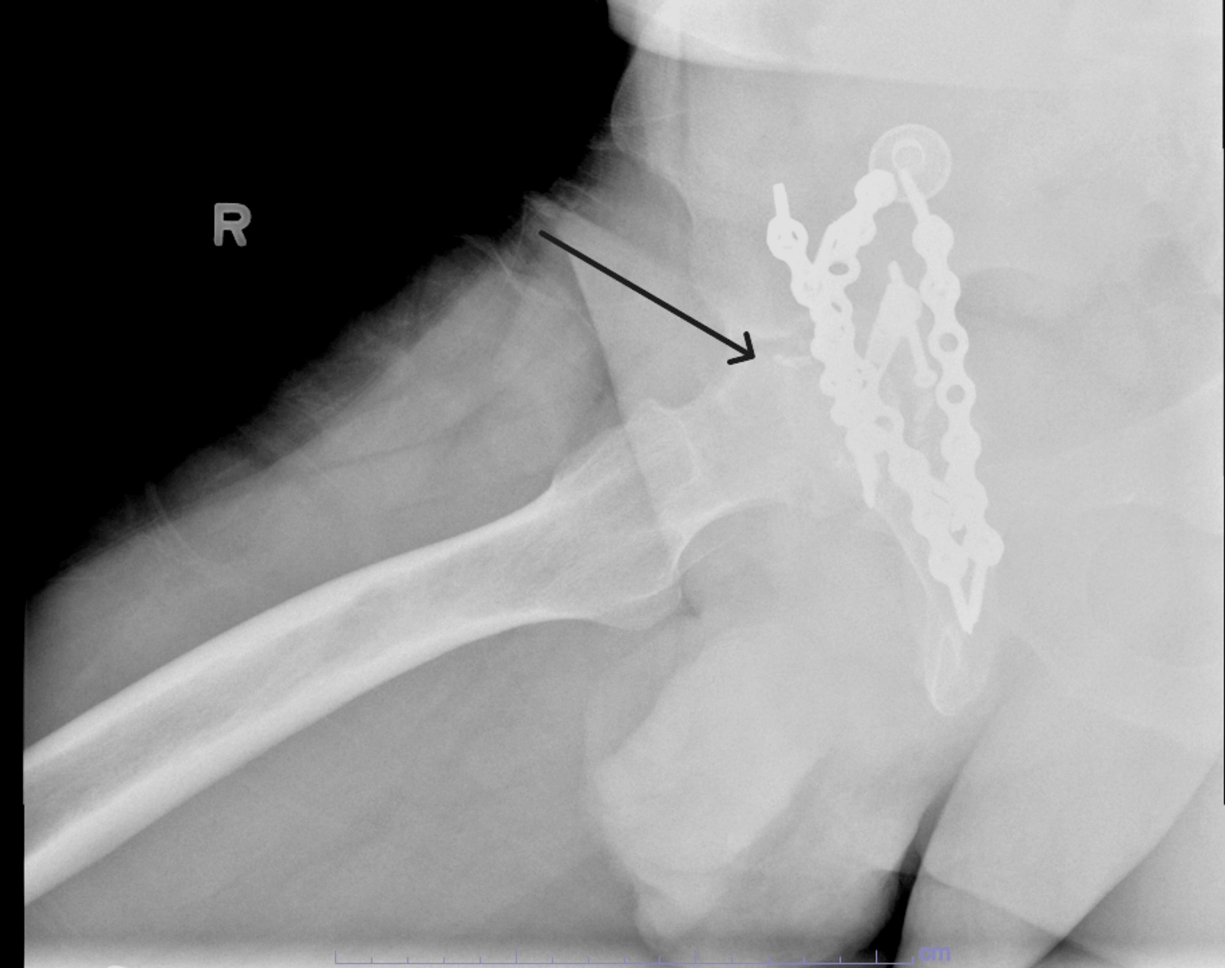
Cross-table lateral X-ray demonstrating a femoral neck fracture with acetabular plating Arrow indicates a femoral neck fracture
